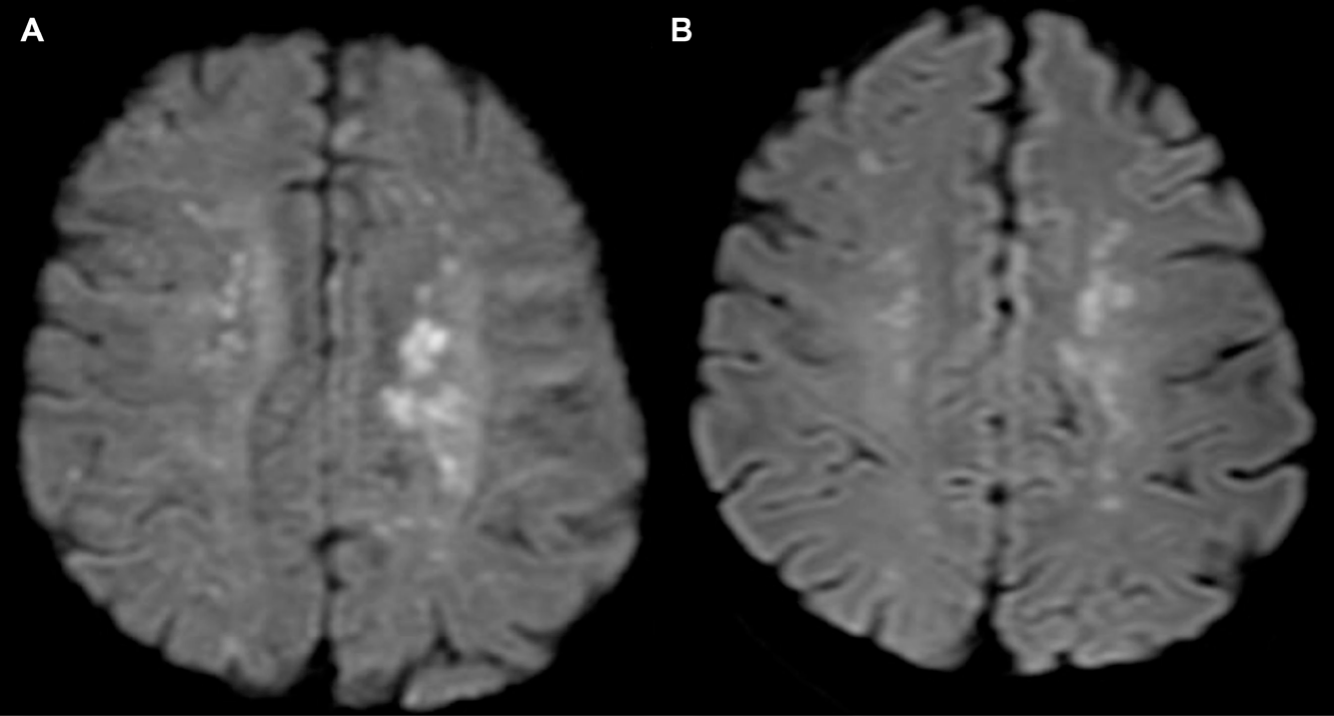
DW-MRI imaging of the brain Images were acquired after acute cerebral infarction (A) and 11 days after the onset of treatment with gefitinib together with anticoagulant therapy (B).
